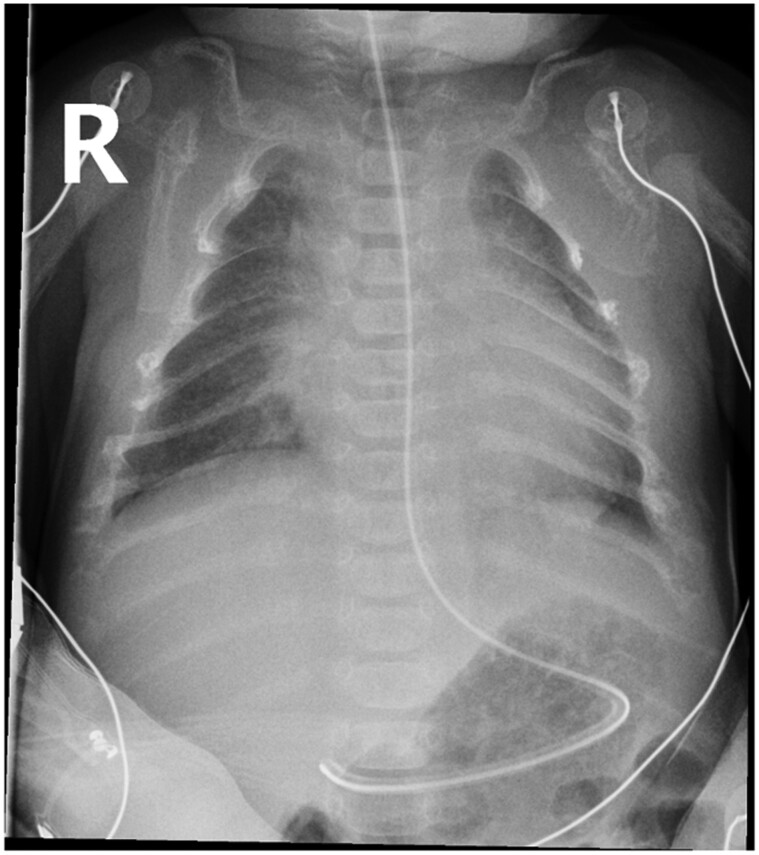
Chest x-ray at presentation showing diffuse osteopenia and coarsened trabeculae throughout the thoracic cage.
